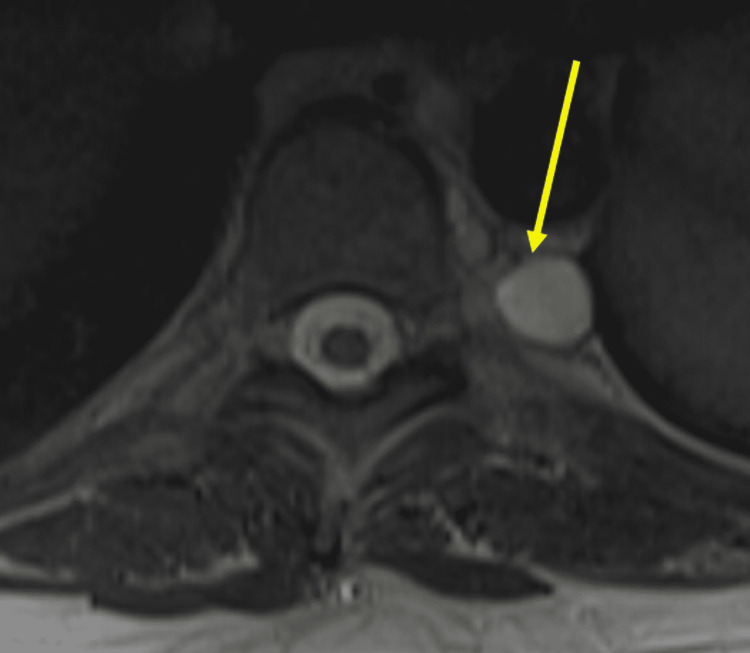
Full-body MRI showing the largest nerve sheath tumor at the level of T7 T2 hyperintense paraspinal non-enhancing lesions on the left from levels T4-T8. The largest is at the T7 level and measures 2.0 x 1.4 cm, previously 2.2 x 1.9 cm.
